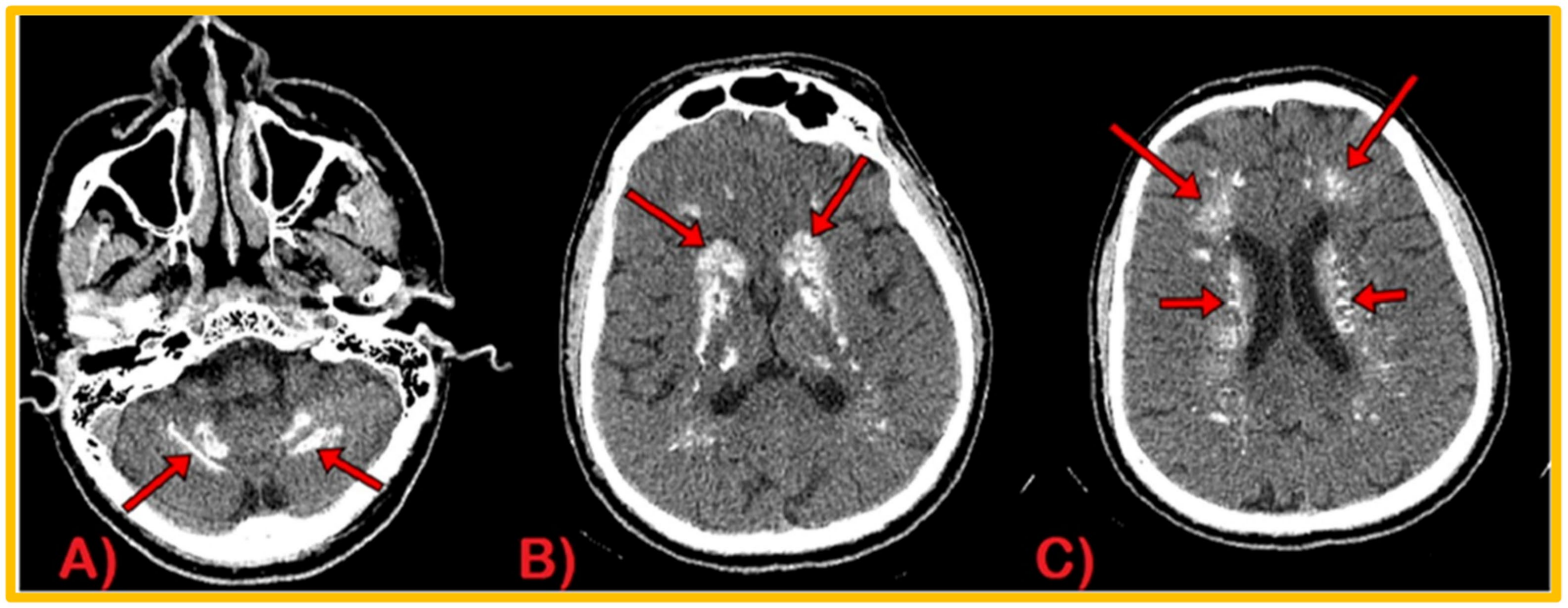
CT Brain Plain Axial views: calcification in dentate nuclei (A), basal ganglia (B), and periventricular and semiovale regions (C).
